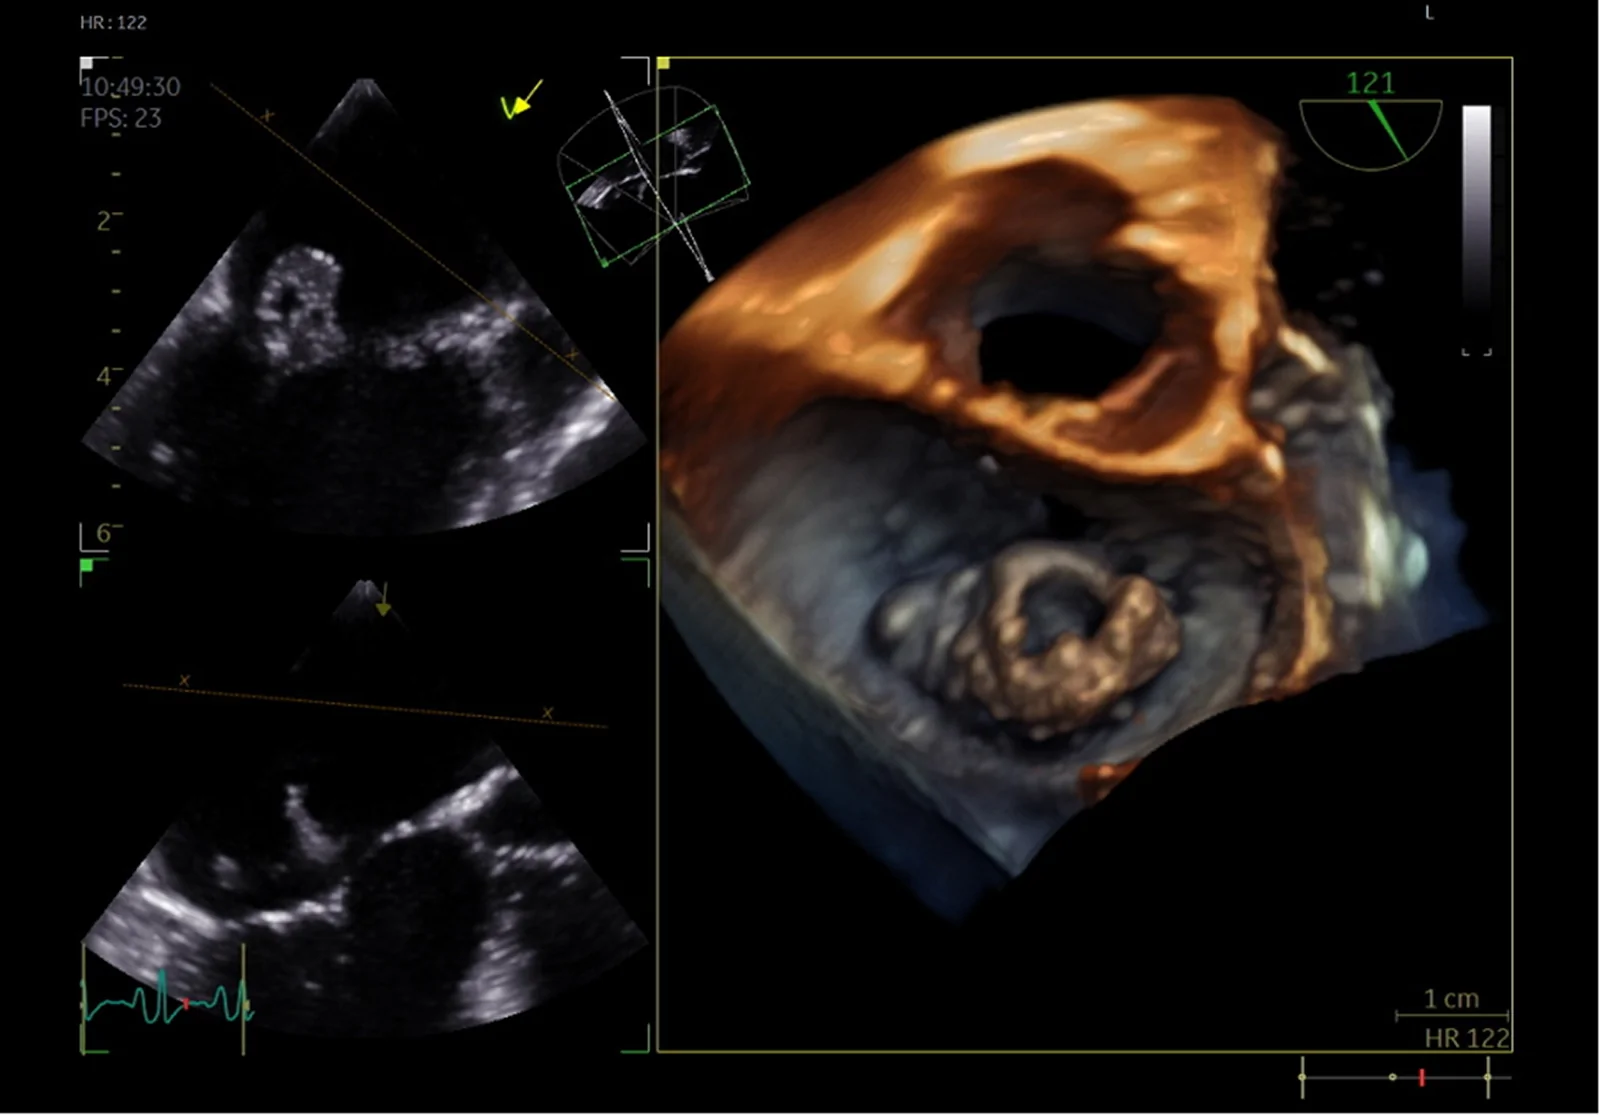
Transoesophageal echocardiography demonstrating large vegetation on A3 scallop of mitral valve. (Patient 2).
